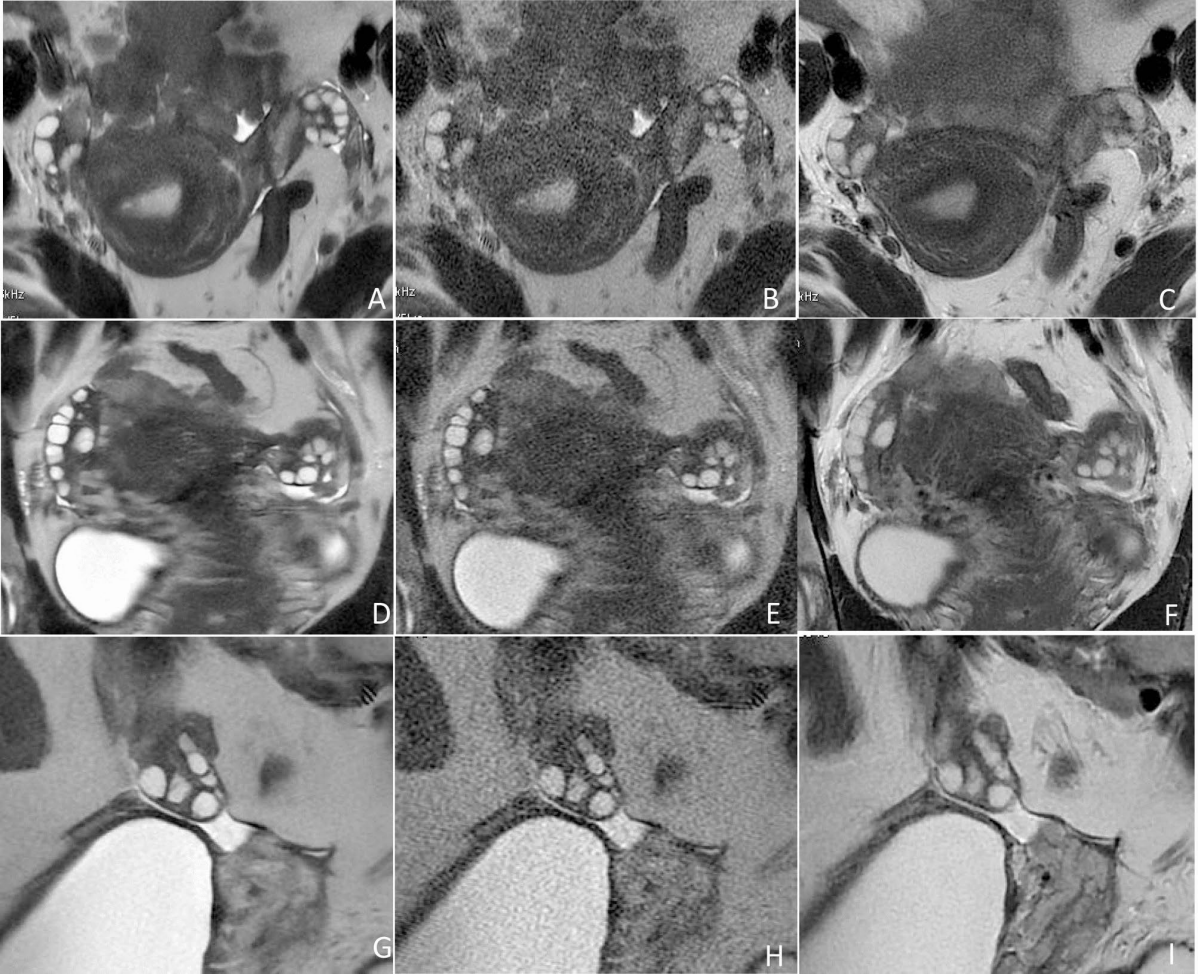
Representative T2-weighted MR images of the ovaries in a 24-year-old woman with PCOS. The SSFSE-DL images (A, D, G) clearly show bilateral ovaries and follicles with minimal noise and blurring artifacts, providing optimal conspicuity of follicles. In SSFSE-C images (B, E, H), follicle conspicuity is primarily influenced by noise, whereas in PROPELLER images (C, F, I), it is predominantly affected by motion-related blurring artifacts.
